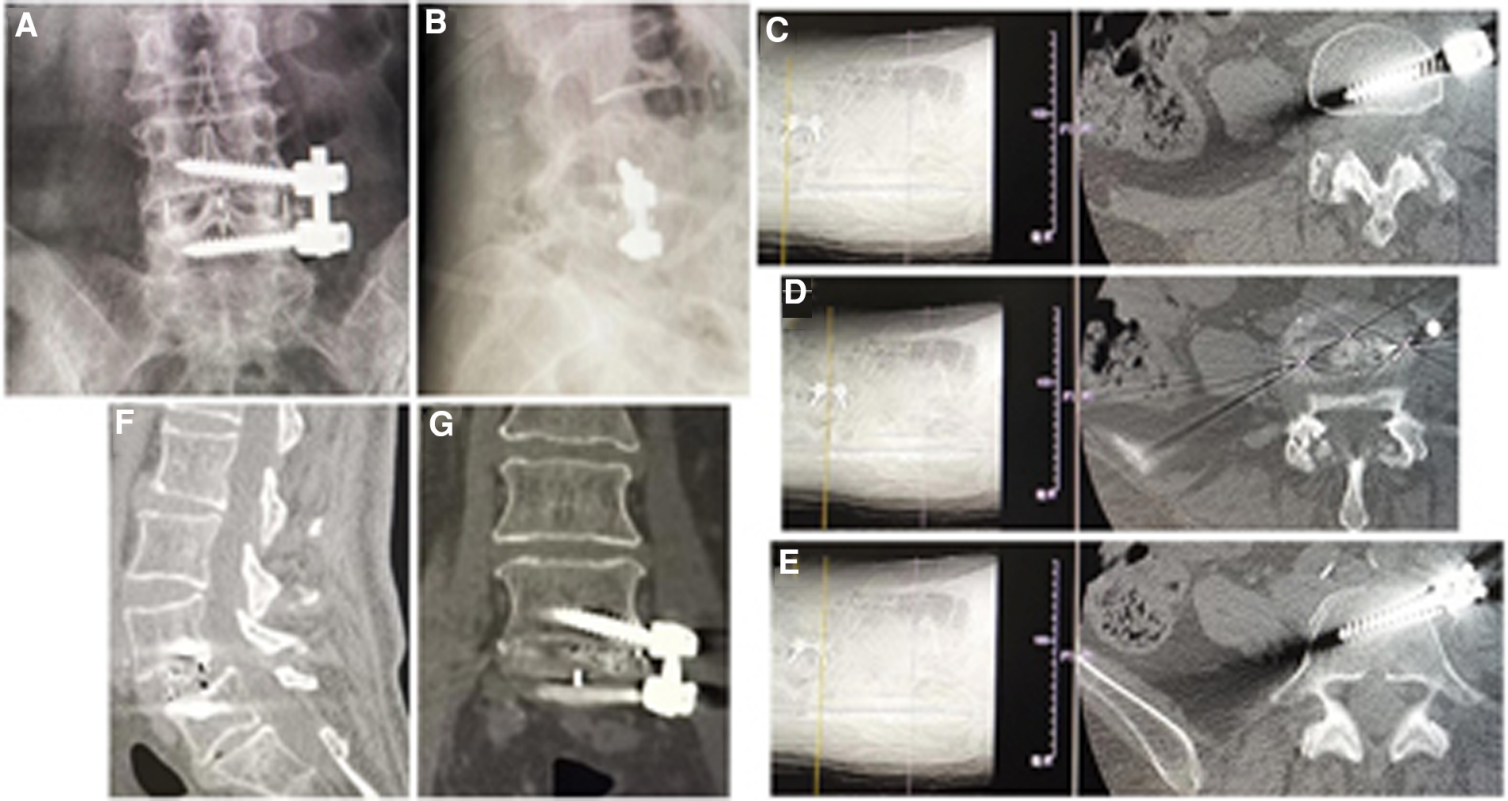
(A) Posteroanterior and (B) lateral x-ray image, and (C–E) axial CT images showed good position of cage and screws after operation. Fusion grade at 2-year follow-up was grade I on (F) sagittal and (G) coronal CT image.
